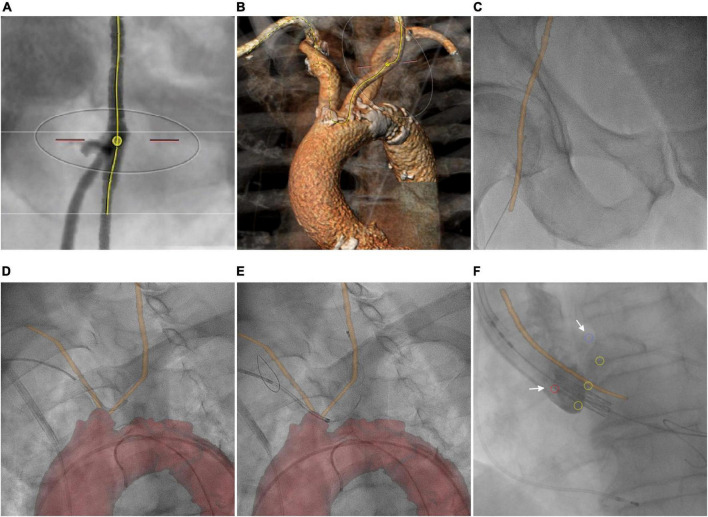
Transcatheter aortic valve replacement. (A) CTA-based determination of the transfemoral puncture site. (B) Volume rendering shows the route for implantation of the cerebral protection device. (C) Overlay of the femoral centerline on fluoroscopy. Image fusion of the patient-specific vascular centerline (orange line) during (D) and after (E) implantation of the cerebral protection system. (F) Depiction of aortic cusp hinge points (yellow circles), coronary ostia (red and blue circle, arrows), and aortic centerline (orange line) during valve implantation.
